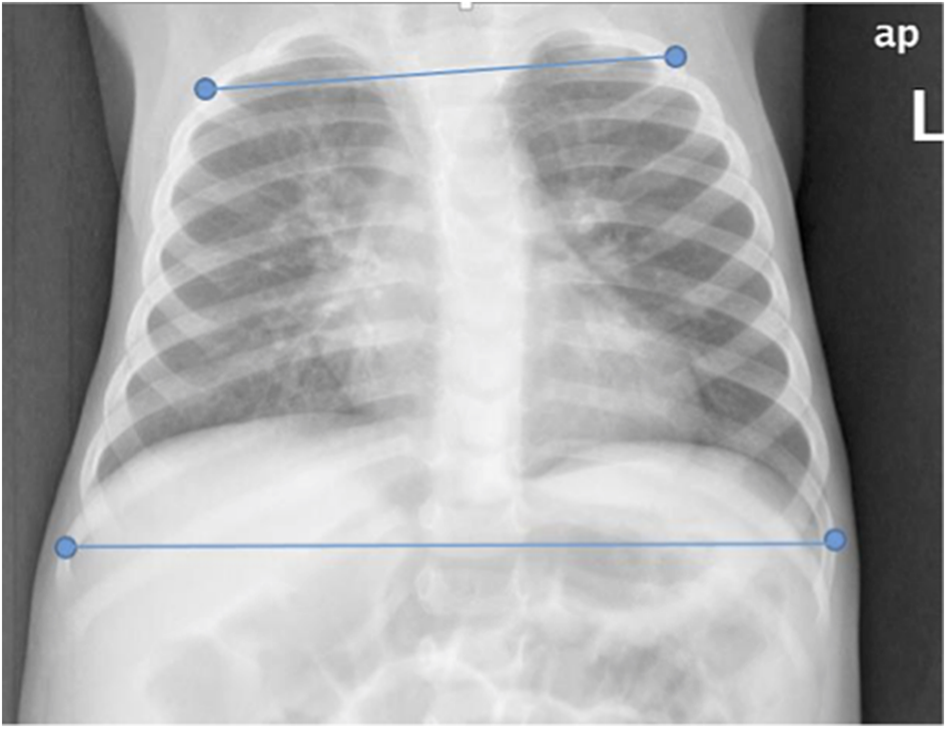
Chest x-ray with markings on the edges of the 2nd and 9th ribs for distance measurements. Similarly, we also measured distances at edges of the 4th and 8th ribs.
